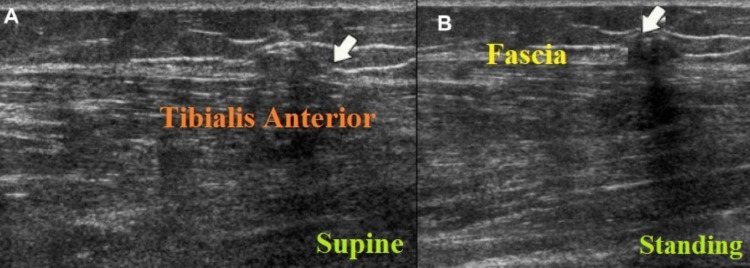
Longitudinal high-resolution ultrasonography images Longitudinal high-resolution ultrasonography image of the tibialis anterior muscle in supine position (arrow) demonstrating focal fascial defect (A). Longitudinal high-resolution ultrasonography image in weight-bearing standing position demonstrating focal herniation of hypoechoic muscle through the defect, with a mushroom-like appearance (arrow) consistent with features of focal muscle herniation (B).
